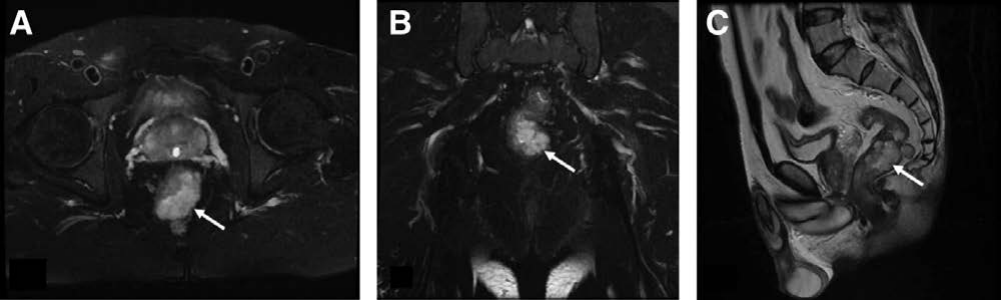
Pretreatment MRI image (T2W1): multiple irregular nodular thickening of the lower rectal wall, showing long T2 signals, and progressive enhancement on enhanced scan (white arrow). (A) Axial view; (B) coronal; (C) sagittal view.
